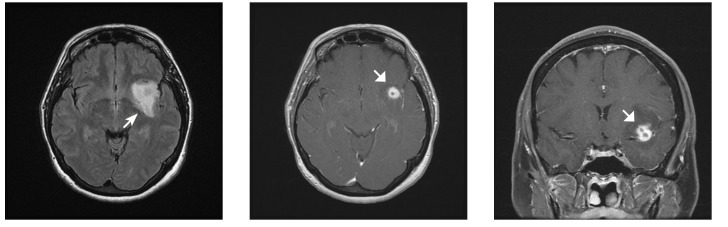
Axial magnetic resonance imaging suggestive of insular glioma, with concerning features including confluent, anatomically restricted fluid-attenuated inversion recovery hyperintensity (arrow) and nodular central enhancement with probable areas of internal necrosis (broad arrows).
